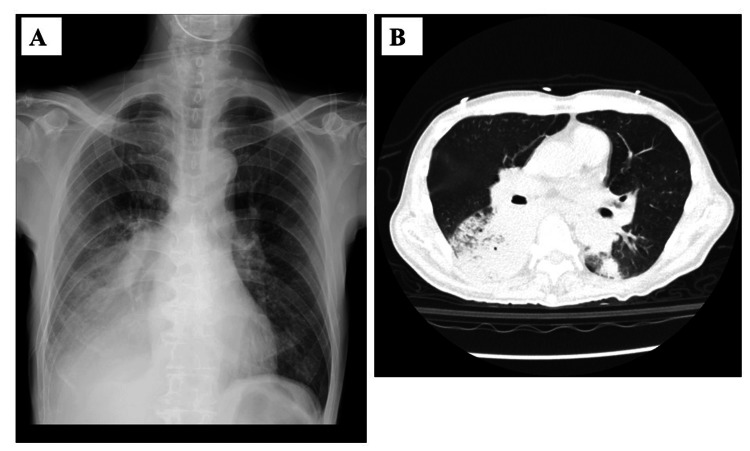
Chest X-ray (A) and computed tomography (B) images of the patient with severe pneumonia and bacteremia due to Legionella pneumophila serotype 3 and co-infection with methicillin-susceptible Staphylococcus aureus (MSSA) and b-lactamase-negative Haemophilus influenzae (BLNAR). Massive infiltration shadows are seen mainly in the right lung and left lower lung.
